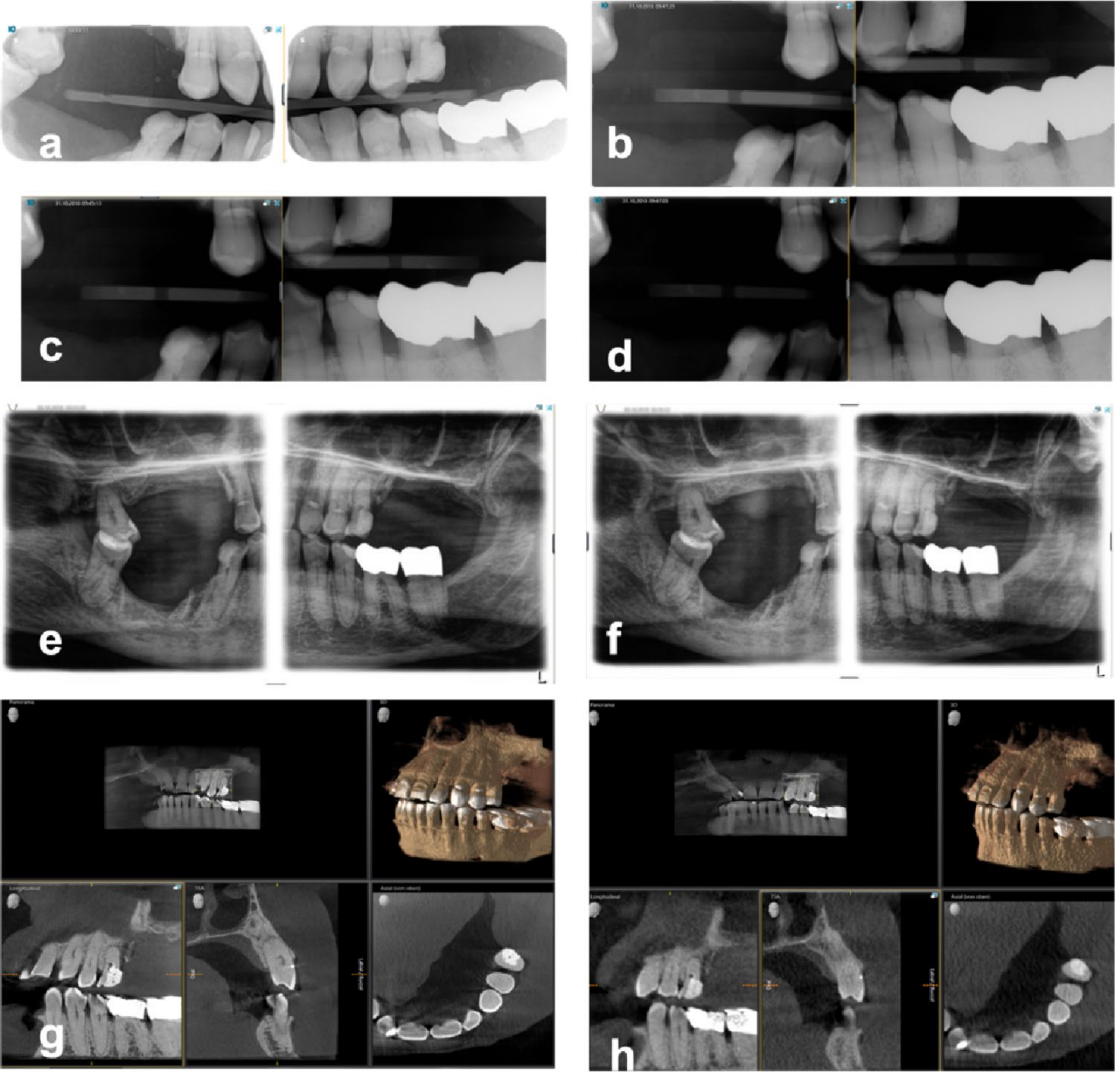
Representative examples of bitewings recorded using the (a) imaging plate (60 kV, 0.32 s) (b) digital sensor (60 kV, 0.08 s), (c) digital sensor (60 kV, 0.16 s), (d) digital sensor (70 kV 0.08 s), (e) BWpan (69 kV, 12 mA), (f) BWpan (72 kV, 14 mA), (g) CBCT HD (85 kV, 6 mA), (h) CBCT LD (85 kV, 10 mA)
